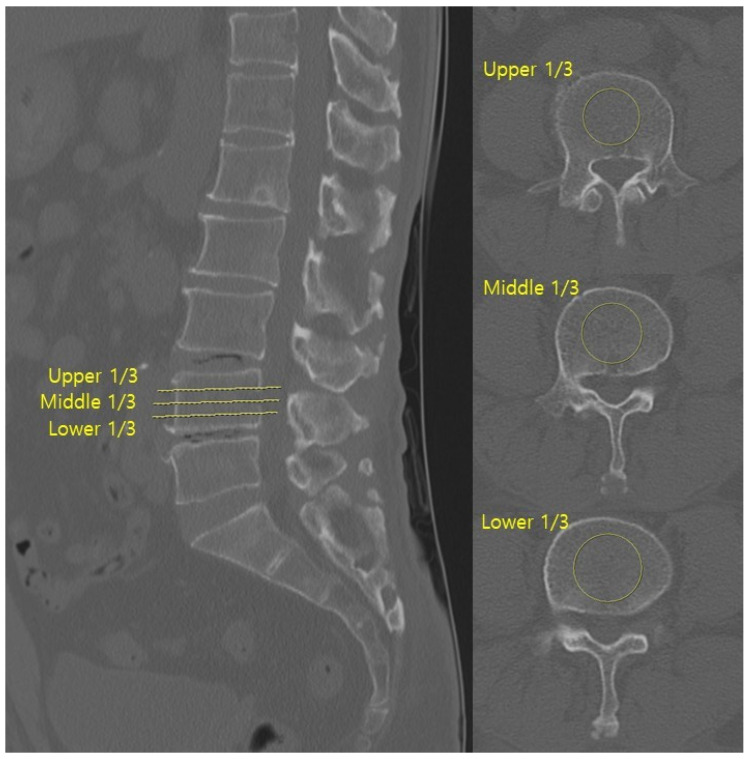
Hounsfield units were measured on axial CT images by averaging the values from three ROIs within the trabecular bone of both adjacent vertebrae at the surgical level.
